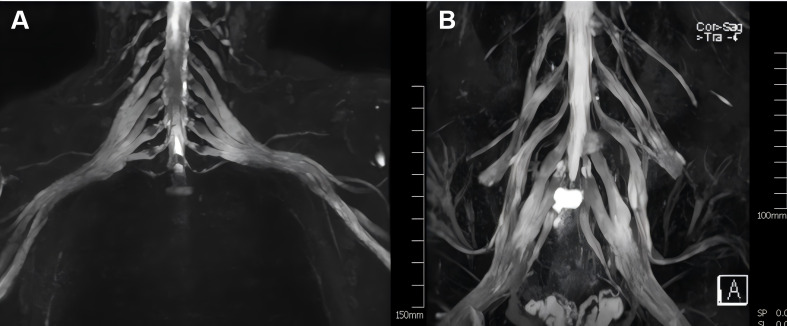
MRI of the brachial and lumbosacral plexuses shows thickening and enhancement of the nerve roots. (A) Coronal T1-weighted fat-suppressed post-contrast image of the brachial plexus shows bilateral, symmetric thickening and marked homogeneous enhancement of multiple nerve roots, involving both the trunks and divisions. (B) Coronal T1-weighted fat-suppressed post-contrast image of the lumbosacral plexus demonstrates similar findings: diffuse hypertrophy and pathological enhancement of the cauda equina and lumbar nerve roots.
